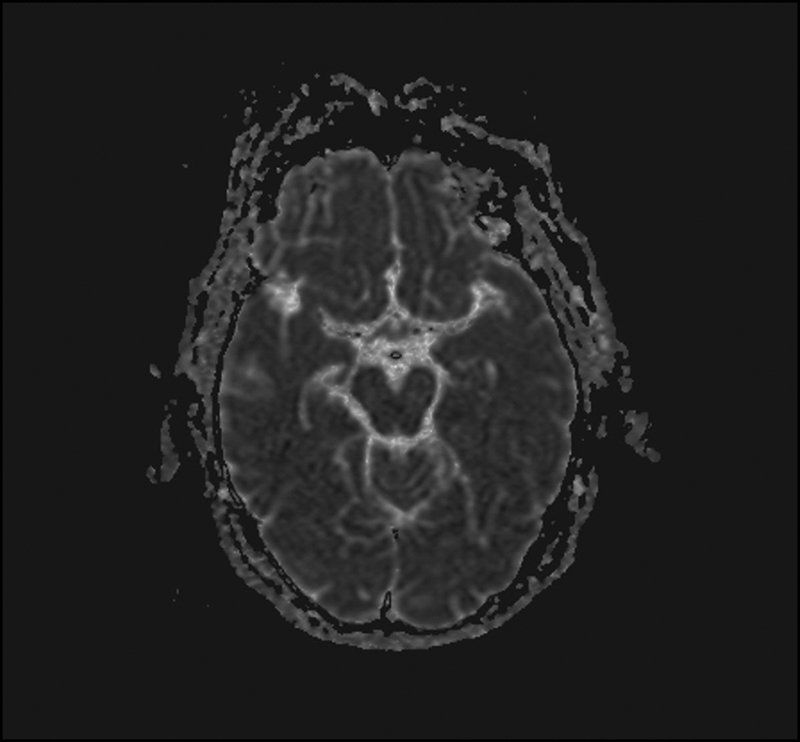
Diffusion-weighted magnetic resonance imaging was normal.
